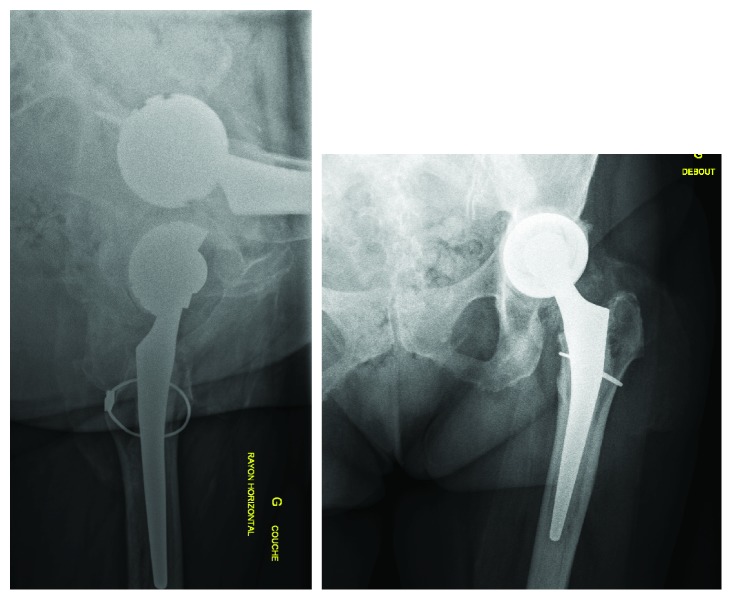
Post revision surgery X-rays at six-month follow-up.
